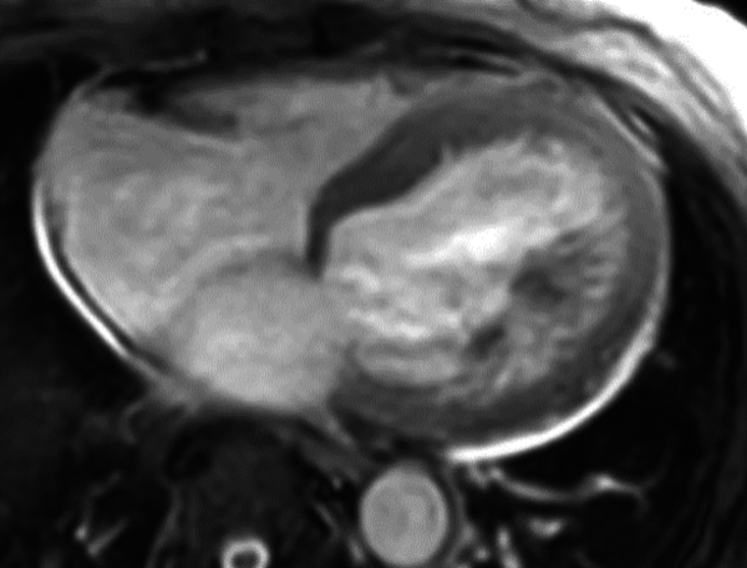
Cardiac Magnetic Resonance Imaging for Patient 3 Showing Patchy Late Gadolinium Enhancement
